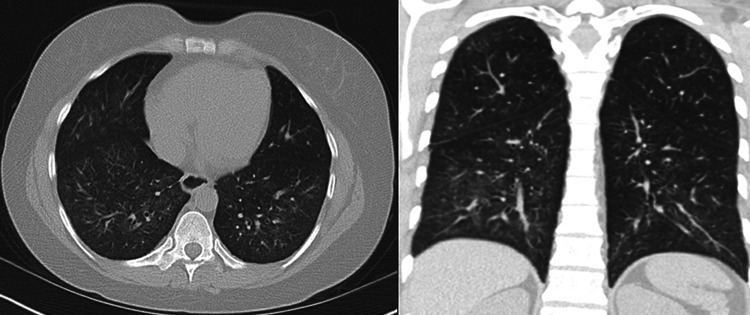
Repeat CT scan of the chest two years after she started on belimumab showing marked improvement of the bilateral lower lobes ground-glass opacity (coronal section on the right and a sagittal section on the left).
